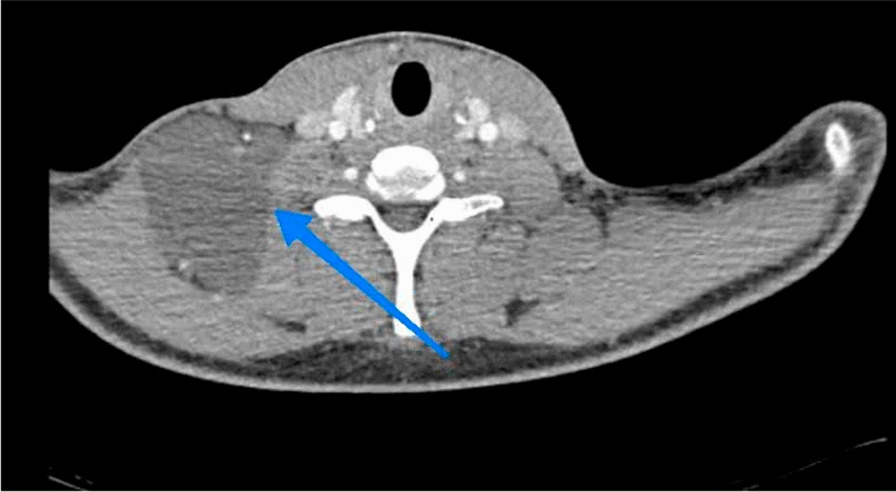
Transverse view of the neck computed tomography scan. The arrow shows a homogeneous cystic lesion in the right supraclavicular region, closely related to the vasculature of the head and neck
